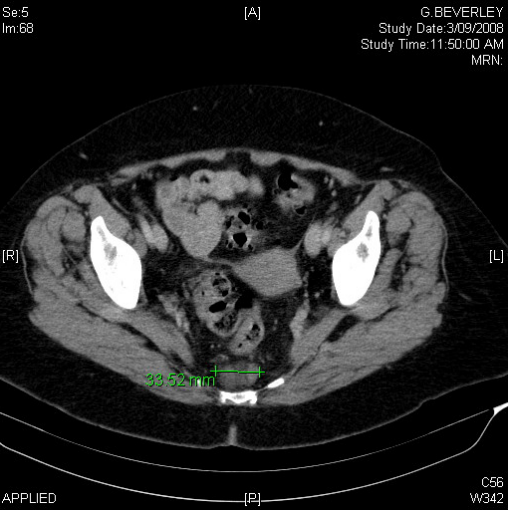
CT transverse view of lesion showing 33.52 mm diameter lesion in the presacral area.
